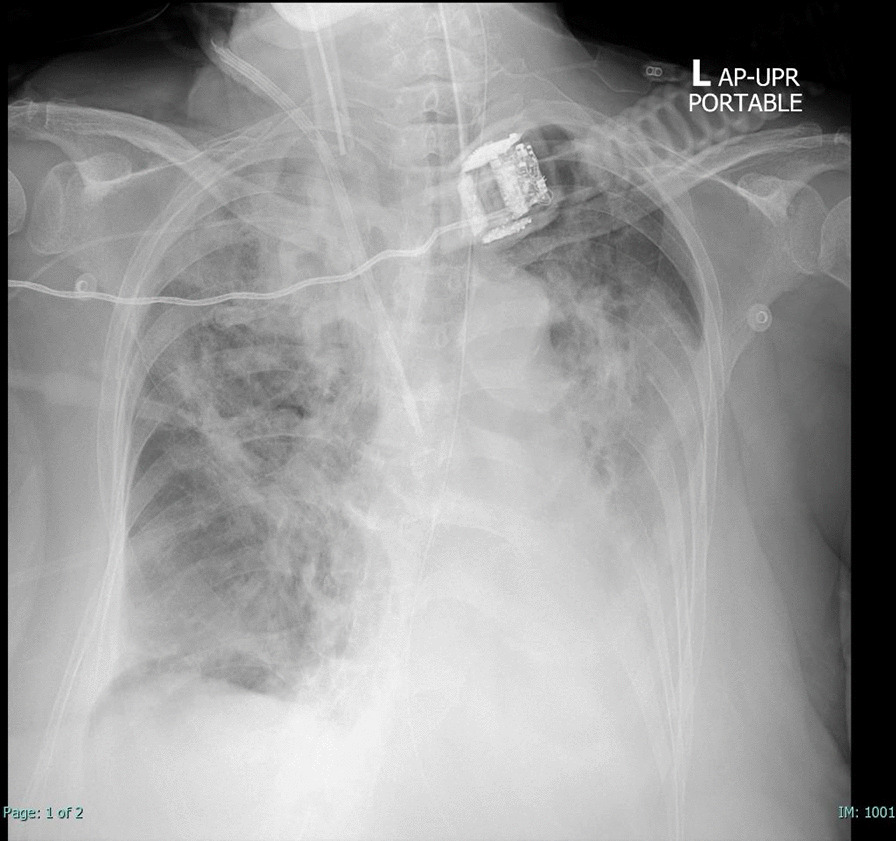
Plain radiograph of the chest on day 11 of hospital admission. Opacity at the right upper and left lower lung fields can be observed
